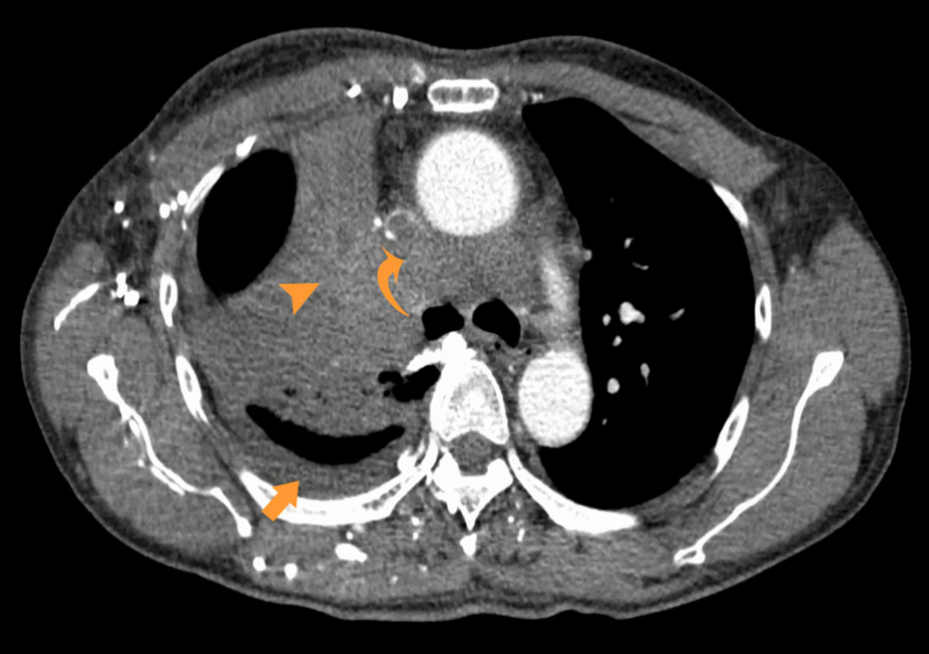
Axial contrast‐enhanced computed tomography (CT) of the chest shows the invasive right lung tumour (arrowhead) causing obstruction of superior vena cava (SVC; curved arrow). Note the presence of pleural effusion (arrow).
